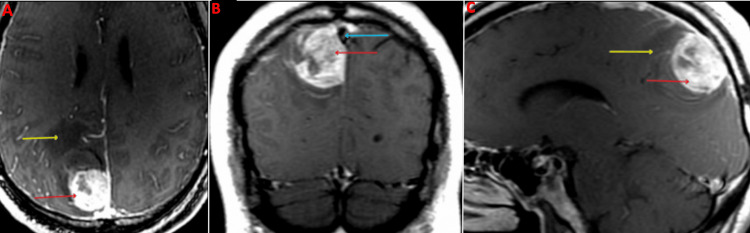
Preoperative MRI brain post contrast with views of the axial (A), coronal (B), and sagittal (C) planes Red arrows: Mass, Blue arrow: Superior sagittal sinus, Yellow arrows: Vasogenic edema
